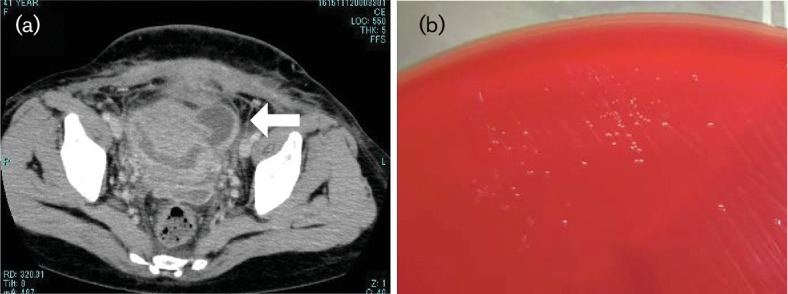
(a) Enhanced computed tomography image showing an abscess around the endocervix (white right arrow). (b) Culture characteristics of *M. hominis.* Pinpoint, translucent colonies are visible on blood agar.
